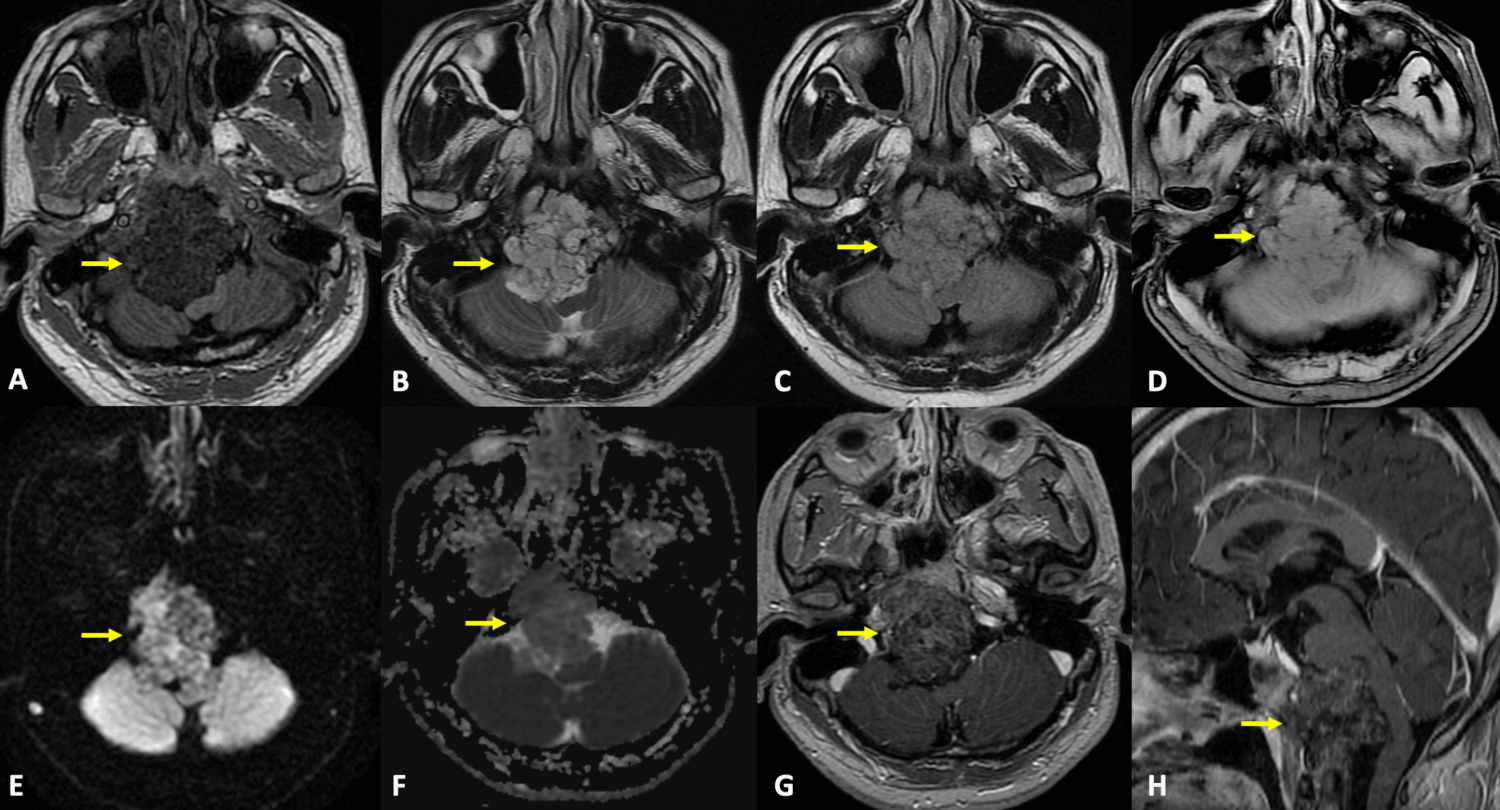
Preoperative MRI with axial and sagittal reconstructions In the clivus region, an irregularly shaped image with partially defined margins and a heterogeneous appearance is observed. On T1-weighted MRI (A), it appears predominantly hypointense compared to the cerebellar gray matter, with linear areas of higher signal intensity. On T2-weighted (B) and fluid-attenuated inversion recovery (FLAIR) (C) sequences, it appears hyperintense with irregular central hypointense lines. The gradient echo sequence (D) shows areas of signal deflection, while the diffusion-weighted sequence (E,F) reveals discrete areas of restricted diffusion. Following contrast administration, there is a heterogeneous enhancement (G,H). This image is associated with erosion of the clivus, right occipital condyle, and petrosal portion of the ipsilateral temporal bone. It extends caudally to contact the odontoid process of the axis and extends superiorly to involve the roof of the nasopharynx and posterior fossa, resulting in compression and displacement of the brainstem (thumb sign). The tumor was marked with a yellow arrow.
